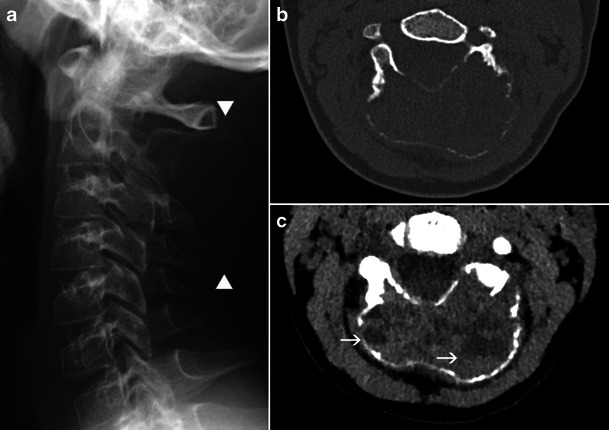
Aneurysmal bone cyst of the second cervical vertebra (C2): a Lateral X-ray of the cervical spine: large expansile lytic lesion of the posterior arch C2 (*between arrowheads*). b CT, axial slice, bone windowing: confirmation of the lesion, with extreme thinning and focal interruption of the bone cortex. c CT, axial slice, soft-tissue windowing: fluid-fluid levels (*arrows*) are identified inside the cyst
